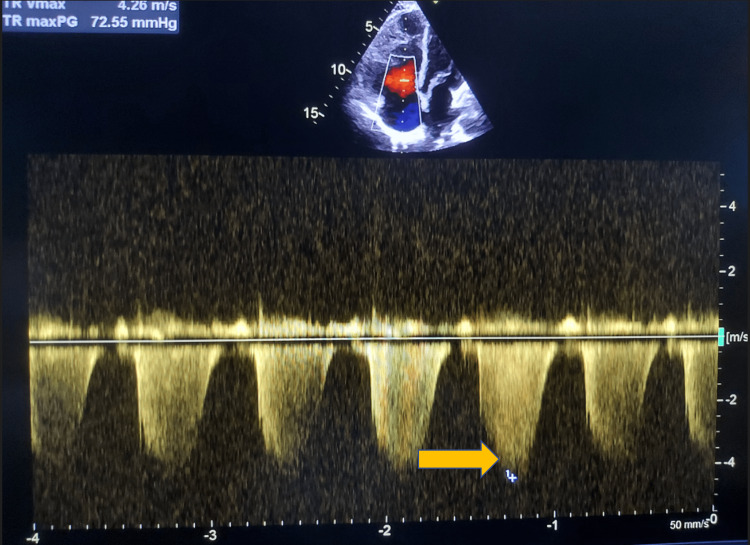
Color doppler echocardiography shows a decrease in TR velocity jet (yellow arrow) after six months of treatment TR: tricuspid regurgitation
